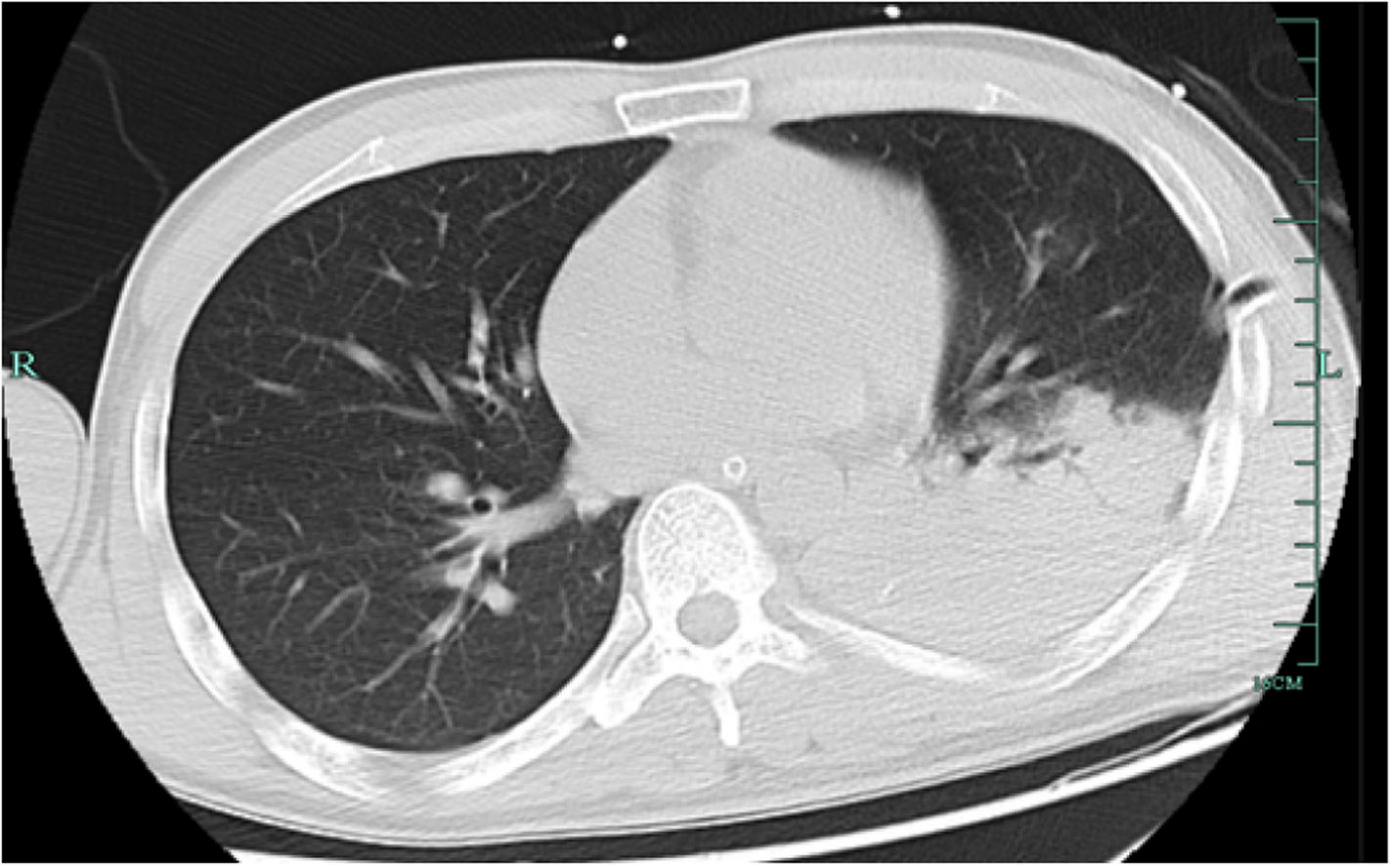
Lung CT scan on November 27, 2018. Lung CT taken on day 3 shows patchy consolidation in left inferior lobar
